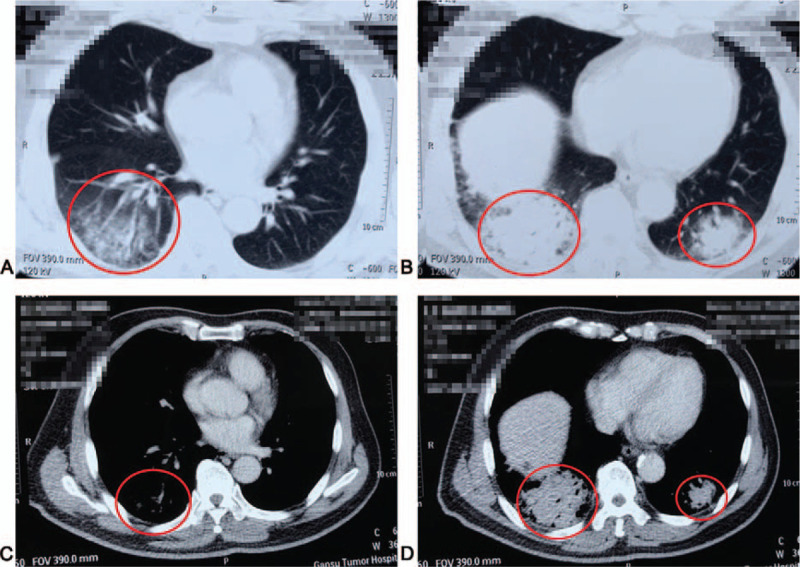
(A–D) CT demonstrated flocculent, cotton-like, high-density shadows (red circle) in the lower aspects of both lungs.
